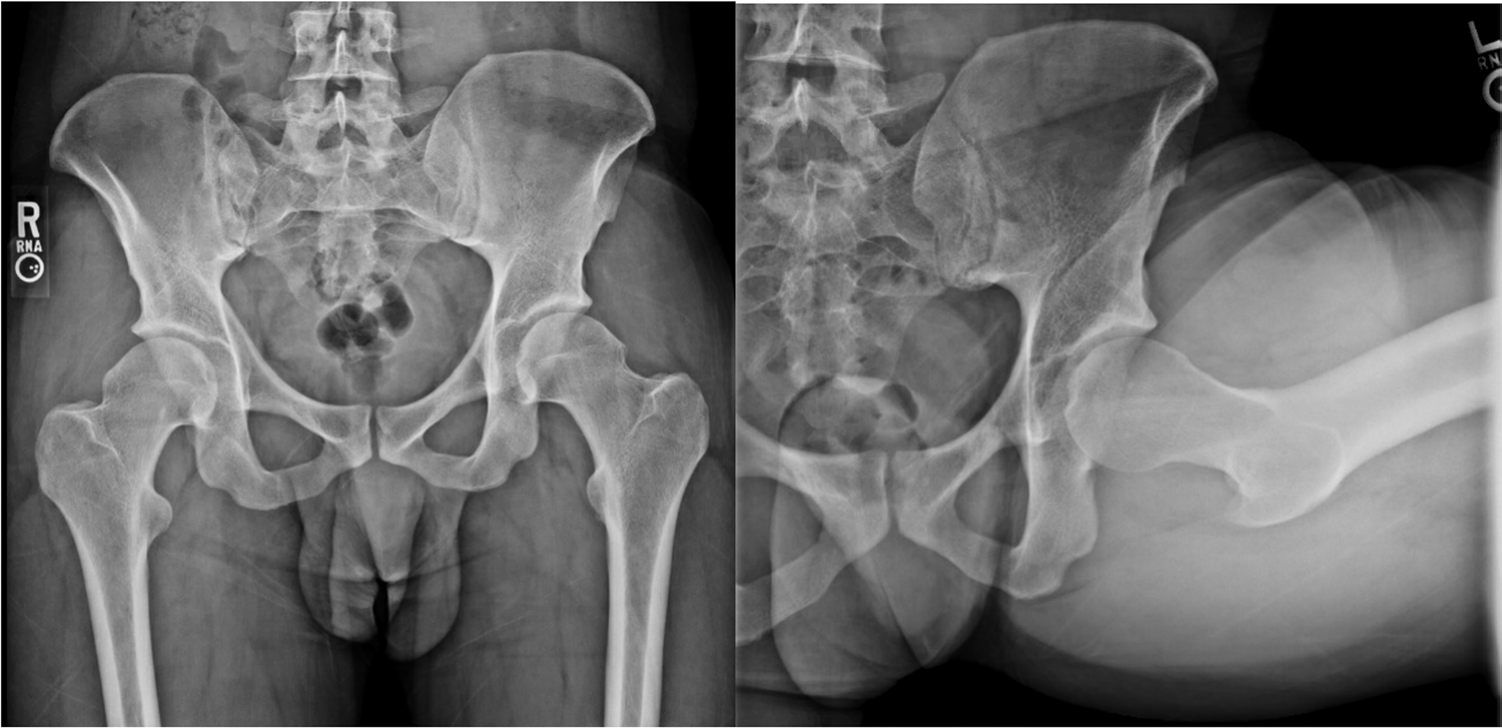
AP and Dunn lateral X-ray views demonstrating a classic cam deformity in a 29-year-old male with symptomatic FAIS. The alpha angle on this hip is 71° on the left with a Tonnis grade 1, Kellgren-Lawrence grade 1, and minimal osteophytic lipping
